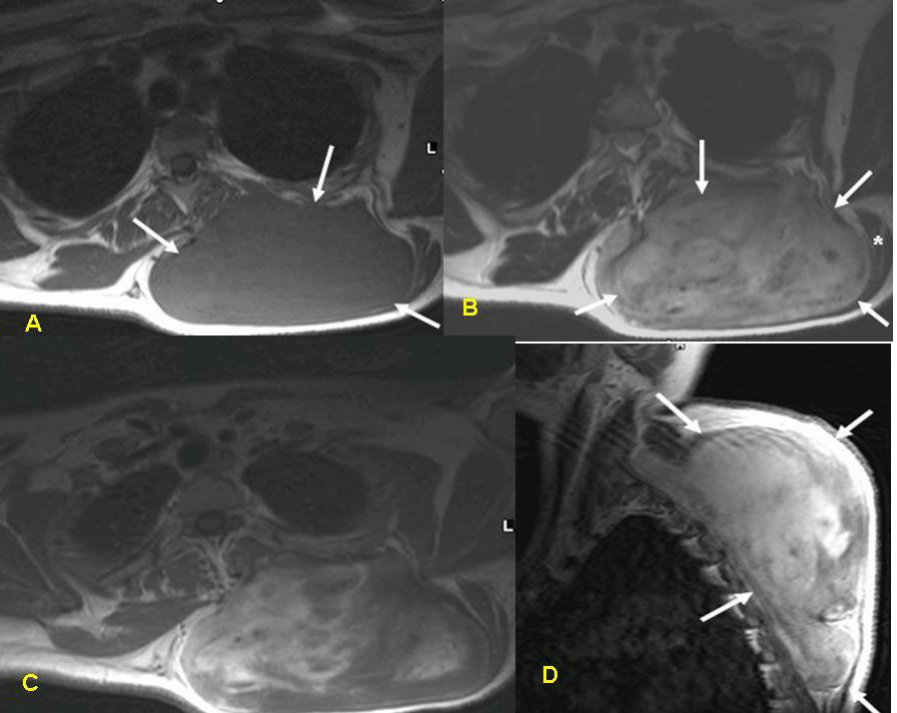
MRI of the tumor: T1W pre-(A) and post-(B) gadolinium injection, T2W (C) and T1W post gadolinium, sagittal view (D). The tumor (arrows) has a heterogenous appearance on T2W images and enhances with the injection of contrast material, demonstrating its vascularity. It is located beneath the trapezius muscle (asterisk) which is atrophic. The paraspinal muscle is compressed medially.
